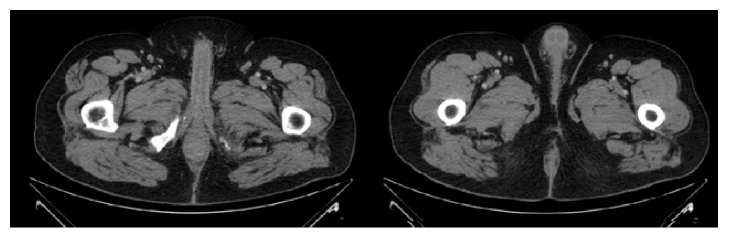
CT scan done 1 year after resection: no evidence of recurrence.
